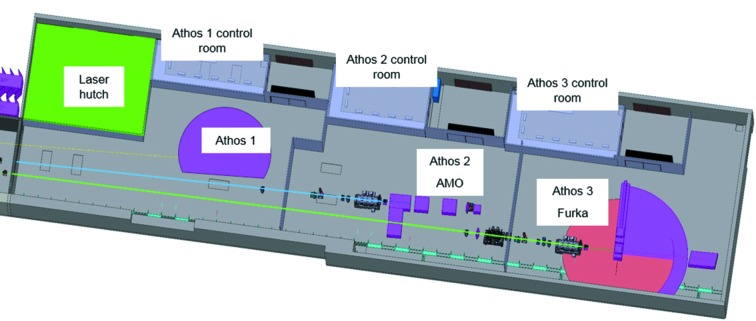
Sketch of the experimental areas of the SwissFEL Athos beamlines. The accelerator is to the left of the above area, with X-rays propagating from left to right.
